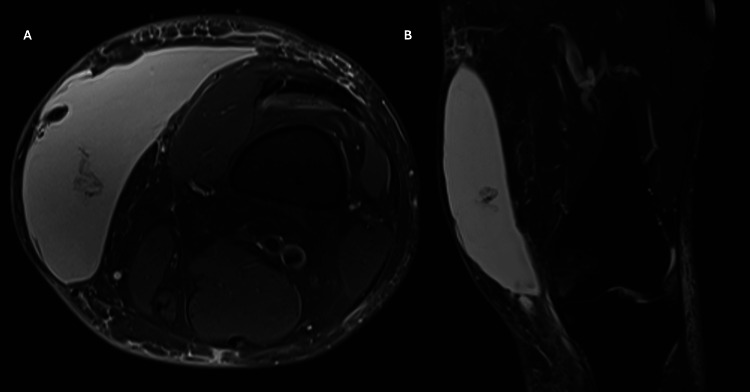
Non-contrast MRI axial view (A) and coronal view (B) of fluid collection
